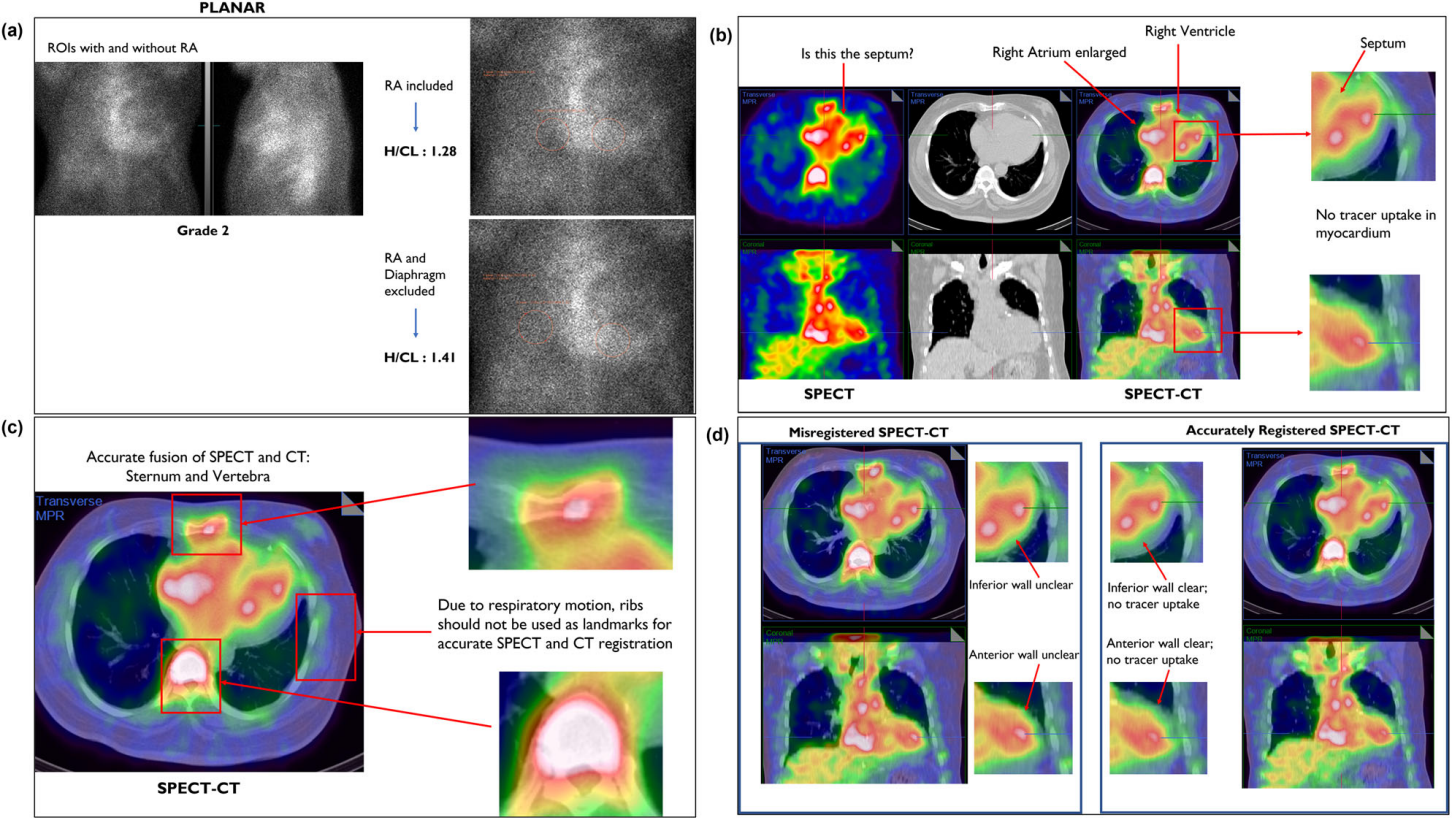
A Three-hour planar imaging demonstrates difference in H/CL ratio calculation when enlarged RA is included in ROI *vs* when RA and diaphragm is excluded. Note lower H/CL ratio when RA is included. B SPECT fails to clearly distinguish between the septum and the enlarged RA and RV. SPECT/CT images clearly show enlarged RA and distinguish septum, RA and RV. SPECT/CT images demonstrates no PYP uptake in the myocardium, changing the classification from strongly suggestive to not suggestive. C Sternum and vertebral body are reference landmarks to accurately fuse the SPECT and CT images. D On the left, SPECT and CT images are inaccurately fused. On the right, SPECT and CT images are precisely fused. With precise registration (on the right), non-tracer avid infero-posterial wall (axial slice, right upper corner) and anterior wall (coronal slice, right lower corner) increase in thickness and are clearly distinguishable as non-tracer avid regions. In the misregistered SPECT/CT images (on the left), the inferior wall appears approximately 3–4 mm; with accurate registration it appears approximately 1.5 mm. Note: the sternum and vertebral body are reference landmarks to be used for accurate registration of SPECT and CT images, which are less effected by the significant inherent respiratory motion. Ribs should not be used as reference landmark given much greater respiratory movement
